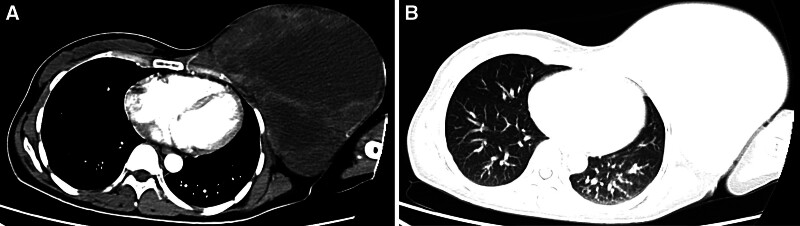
CT image shows a large, slightly dense mass with heterogeneous density in the left breast (A), with mild to moderate heterogeneous enhancement at the edge of the lesion on enhancement scan (B). CT = computed tomography.
